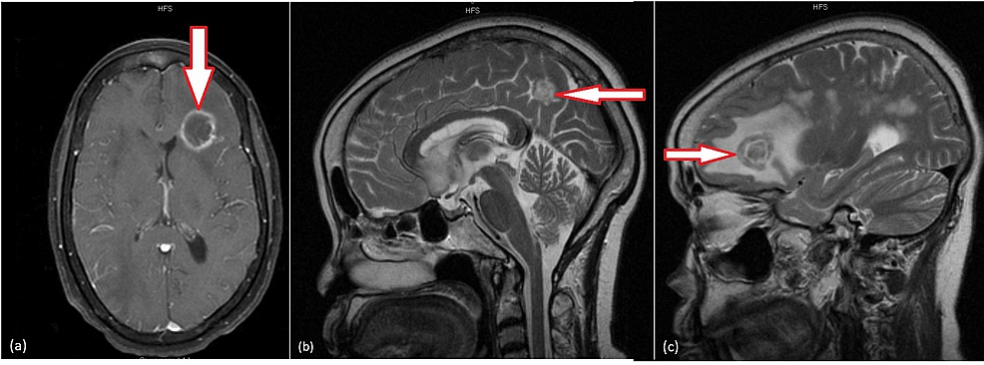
Contrast-enhanced MRI images for Case 2 showing multiple ring-enhancing lesions in the brain, with a large degree of surrounding vasogenic edema. Also observed are concentric alternating zones of hypo- and hyper-intense signals noted on both T2 and T1 sequences, namely “concentric target sign”. (a) and (c) visualize the left frontal lobe lesion sized around 2.5 x 2.1 cm in axial and sagittal views, respectively; (b) is a sagittal view demonstrating a lesion in the right parietal lobe measuring 2.9 x 1.9 cm
